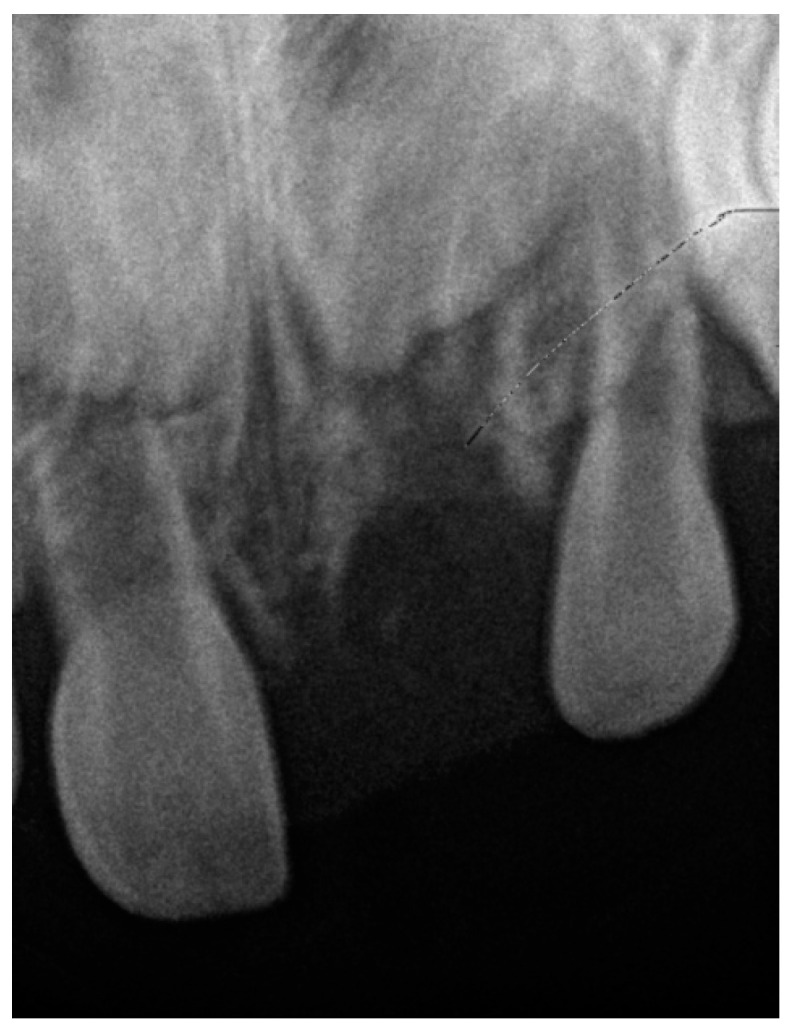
Radiography of traumatized primary teeth in 2016.
